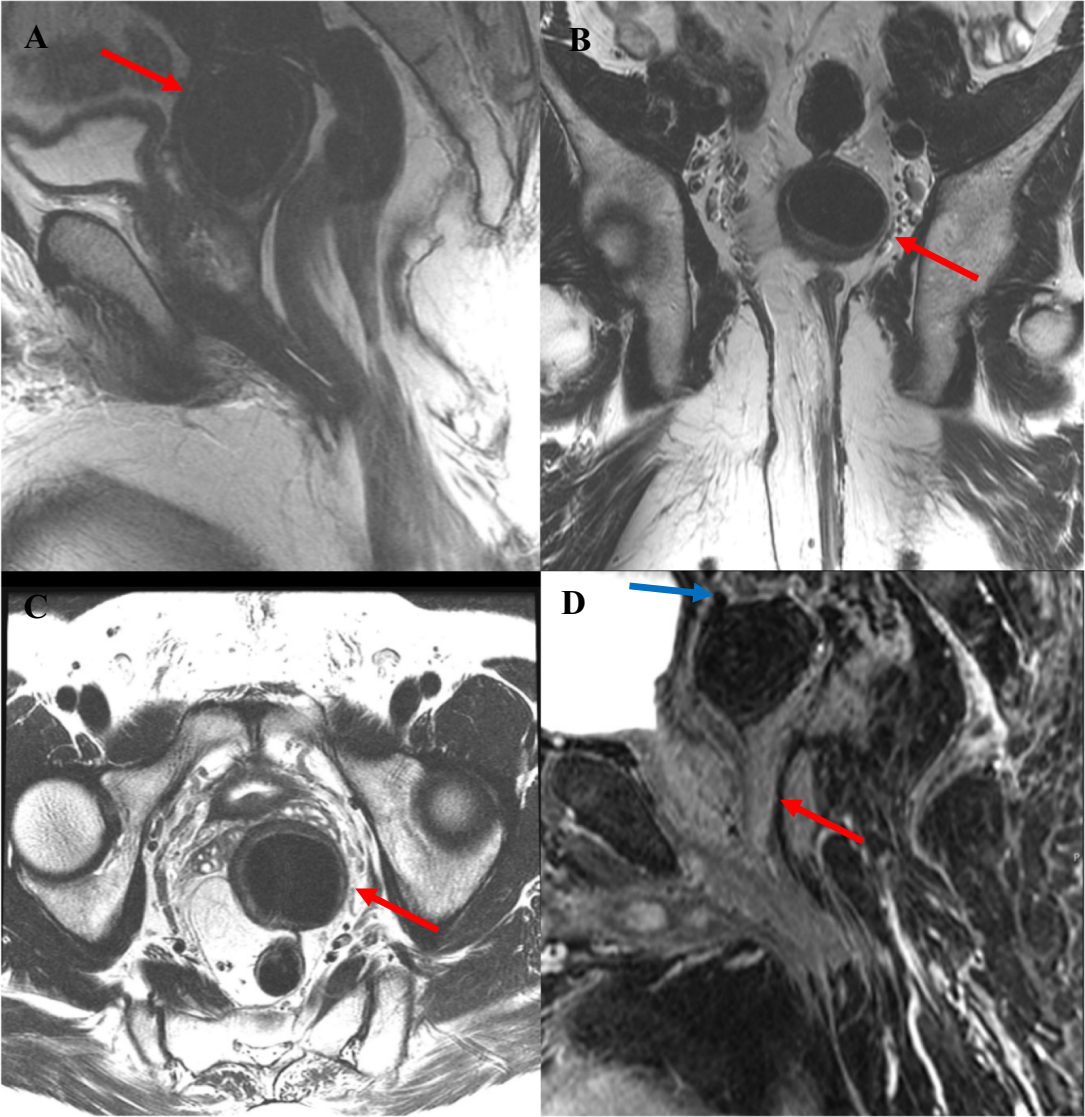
A Preoperative sagittal T2-weighted image showing large thick-walled T2 hypointense structure posterior and superior to the prostate (red arrow) with possible fistula to the sigmoid colon at its superior aspect. There is also a fibrous band or adhesion between the proximal rectum and distal rectum or anal canal. B Coronal image again showing thick-walled mass with adhesion to the distal sigmoid colon (red arrow). This image also demonstrates prolapse or perirectal fat and rectum through the external sphincter. C Axial image showing relationship of rectum, unknown mass (red arrow), seminal vesicles, and bladder. D Sagittal oblique postcontrast images again showing apparent fistula to sigmoid colon (blue arrow) and enhancing tract extending toward the distal prostatic urethra (red arrow)
